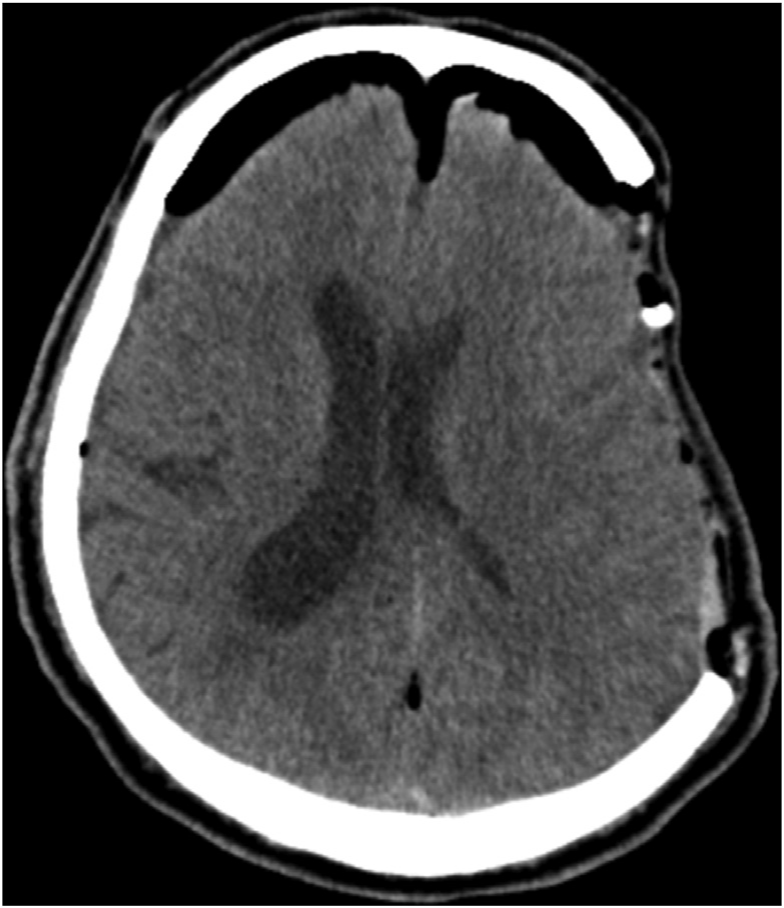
Post-operative brain CT scan, there was no haematoma or midline shift.
